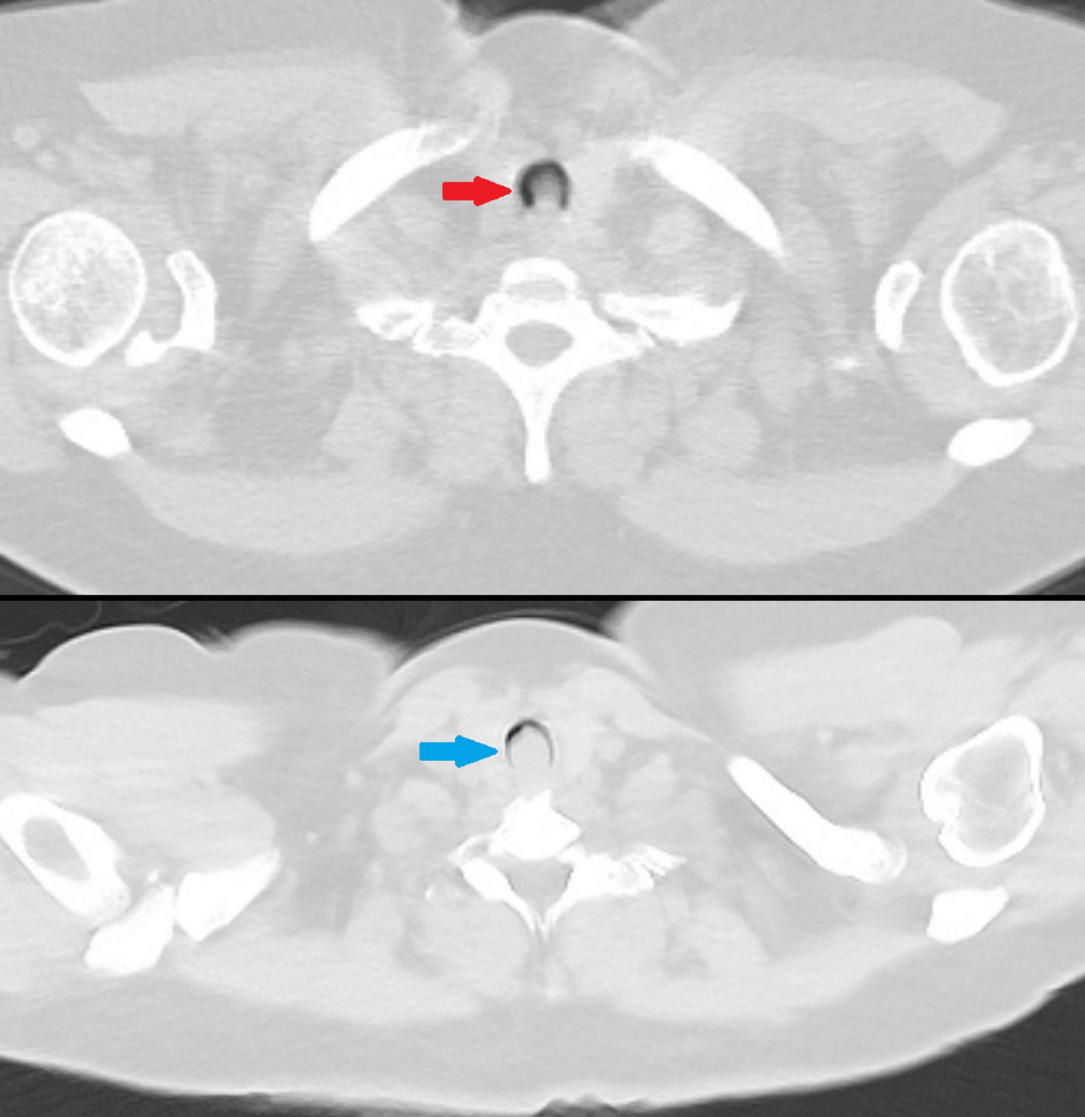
Computed tomography of the chest (lung window, axial cuts) of imaging 5 months prior. Top: depicting tracheal narrowing (red arrow) compared to imaging at the latest hospitalization; Bottom: depicting severe tracheal narrowing secondary to suspected tracheal mass (blue arrow).
